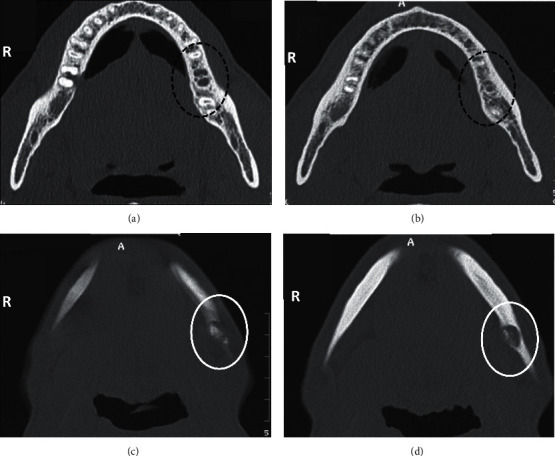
Mandibular axial computed tomography sections in bone window after extraction of the left mandibular second molar (tooth #37). (a) and (b) Sections at the alveolar bone of the tooth #37 showing the absence of bone lesions (black dashed circle). (c) and (d) Sections through the mandibular basilar border allowing the visualization of a mixed image at the region of the left basilar border of 1 cm long axis (white circle).
